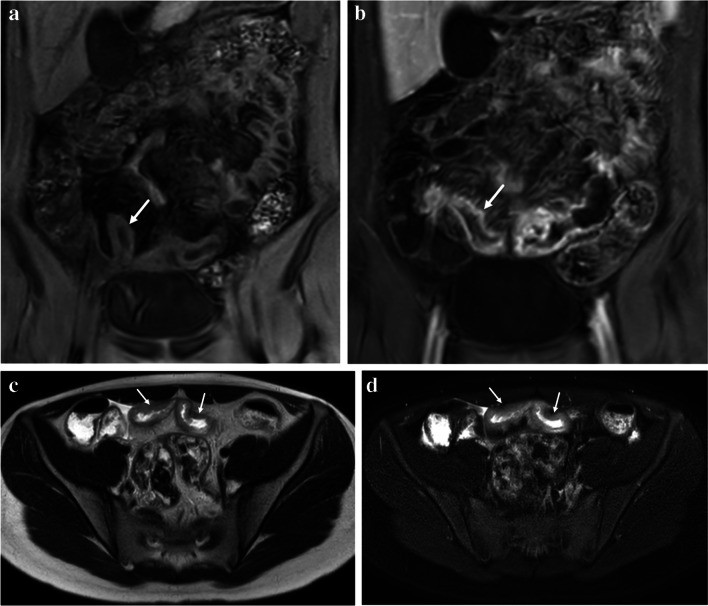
A 17-year-old female with ileal Crohn’s disease (*arrows*). a Coronal unenhanced T1-weighted, (b) coronal contrast-enhanced T1-weighted, (c) axial T2-weighted single-shot fast spin-echo (SSFSE), and (d) axial T2-weighted SSFSE with fat saturation MRI images can be used to calculate the Magnetic Resonance Index of Activity (MaRIA). Components of the MaRIA score include the degree of bowel wall thickening, presence of bowel wall edema, degree of bowel wall postcontrast hyperenhancement, and presence of radiologic ulcers (all of which are present in this patient)
